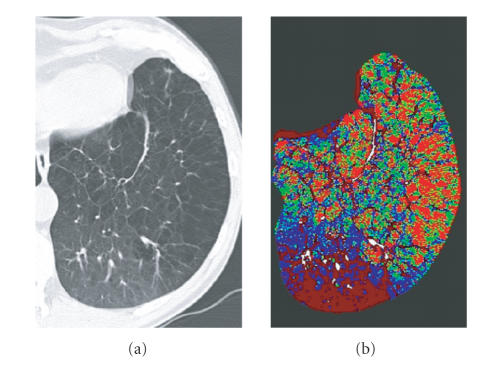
Morphological CT (a) and perfusion map of an emphysematous lung
